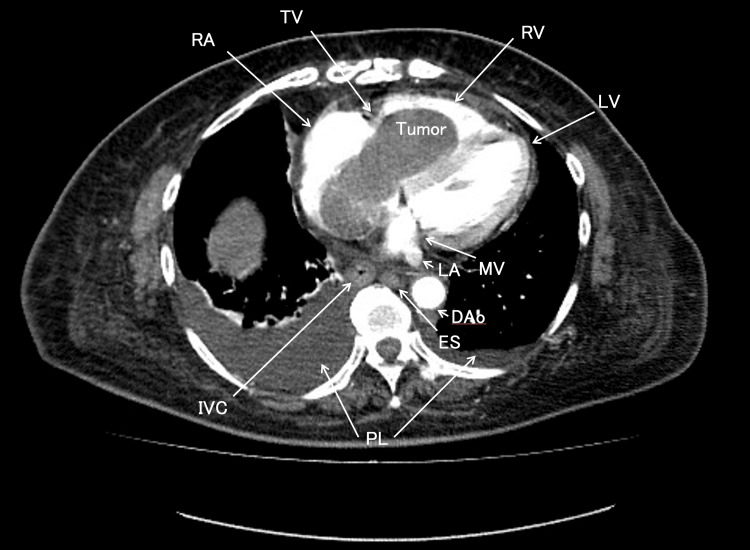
The axial plane of contrast-enhanced early-phase chest computed tomography A giant tumor is entrapped in the tricuspid valve and extends to the right ventricle. A bilateral pleural effusion was observed with right-sided predominance, and the inferior vena cava was filled with tumor. TV, tricuspid valve; RA, right atrium; RV, right ventricle; MV, mitral valve; LA, left atrium; LV, left ventricle; IVC, inferior vena cava; PL, pleural effusion; ES, esophagus; DAo, descending aorta
